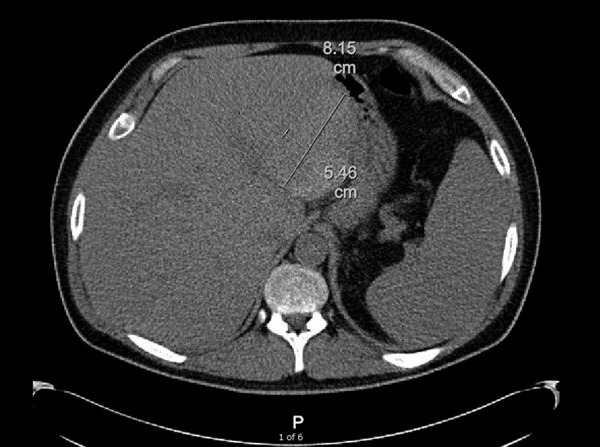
CT-Abdomen demonstrating spontaneous intra-hepatic hemorrhage, measuring 8.15 cm × 5.46 cm.
